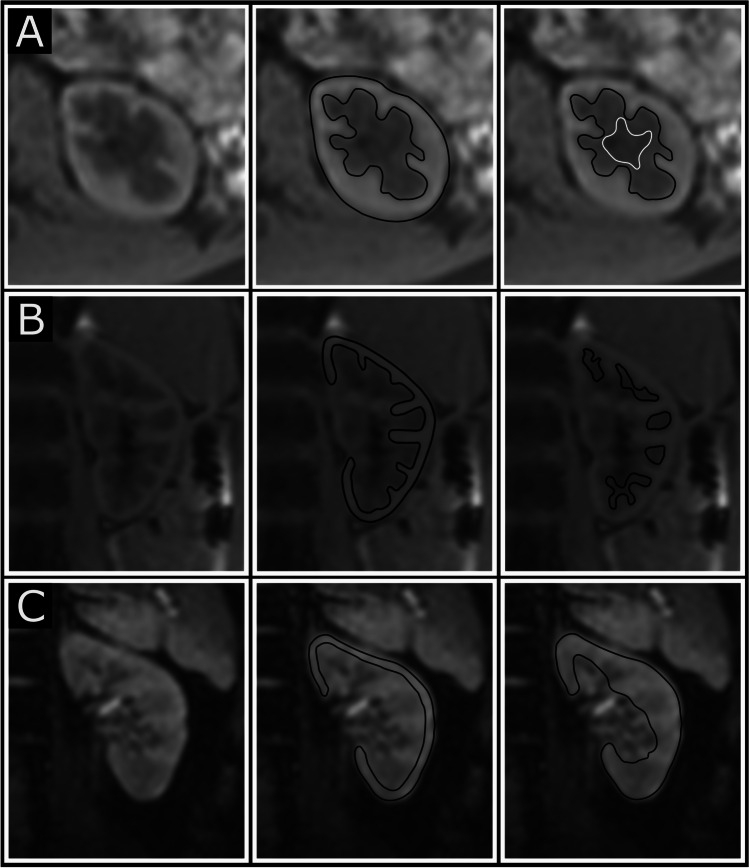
MR images with delineations for renal cortical and medullary volumes (A), and for renal parenchymal T1 (B) and T2* (C) values. A transaxial image of the left kidney for volume measurements without delineations (A; left), and with cortical (A; middle) and medullary delineations (A; right). A coronal image of the left kidney for T1 mapping measurements without delineations (B; left), and with cortical (B; middle) and medullary delineations (B; right). A coronal image of the left kidney for T2* measurements without delineations (C; left), and with cortical (C; middle) and total parenchymal delineations (C; right). Renal parenchymal borders are depicted by black lines, and non-parenchymal tissue removed from volume (A) are depicted by white lines. For T1 and T2* values delineations were placed to avoid partial volume effects. Both left and right kidneys were delineated
